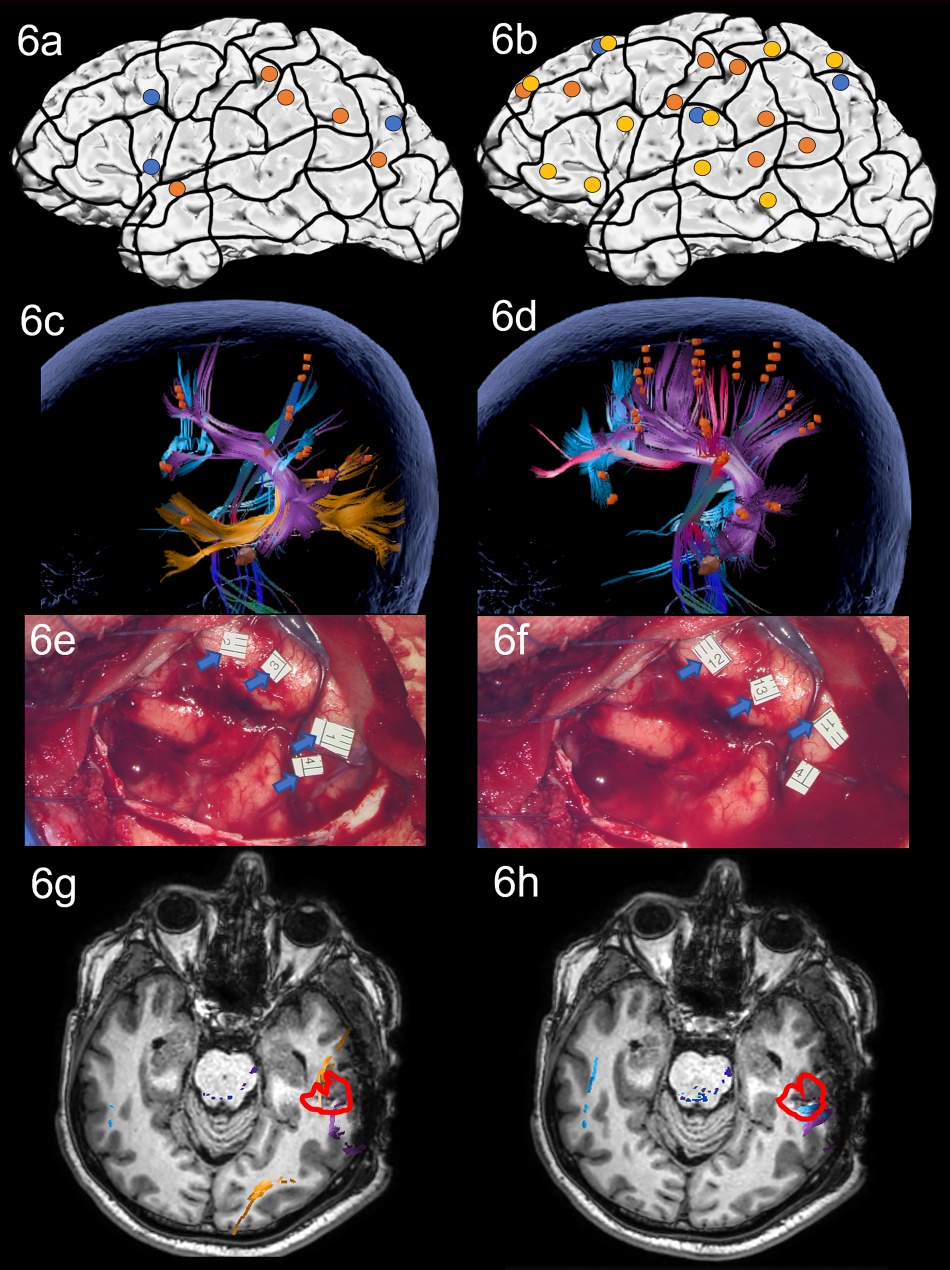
Case 6. Left panels show ON results, right panels AN results. Panels (A, B) nTMS stimulation sites that induced errors; no response and hesitations in orange, semantic errors in yellow and performance errors in blue. Panels (C, D) Fiber tracking results including the lesion, nTMS cortical endpoints in orange and color-coded tracts with CNT in in direction-coded, here dark blue, AF in purple, SLF in pink, ILF in yellow, and CF in light blue. Panels (E, F) Screenshot of intraoperative positive cortical sites with tags 1-3 on the middle and posterior STG and tag 4 on the posterior MTG for ON, and tags 11-13 on the middle and posterior STG for AN. Panels (G, H) Overlap of tracking and postoperative T1-weighted imaging after elastic fusion with the resection cavity marked in red.
